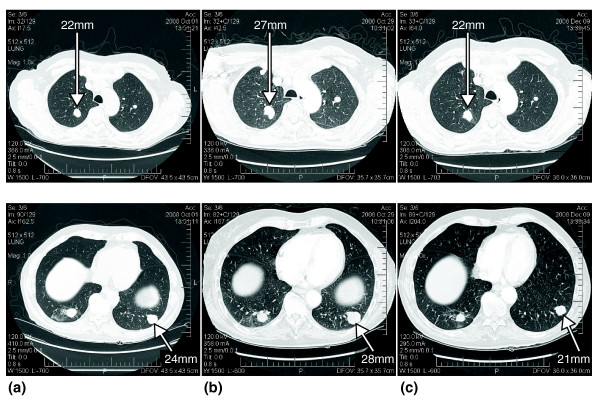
PET-CT scans of the patient. (a) 1 October 2008, 1 month before sunitinib initiation. (b) 29 October 2008, baseline before sunitinib initiation on 30 October 2008. (c) 9 December 2008, 4 weeks on sunitinib.
